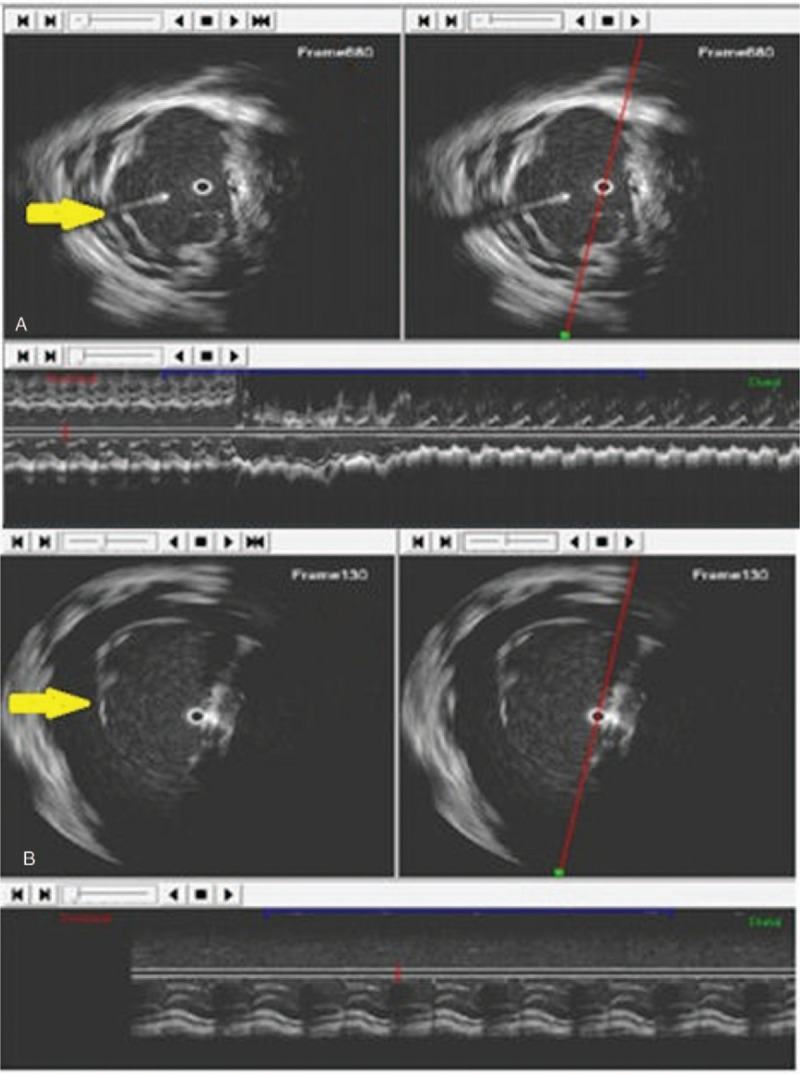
Intracardiac echography using a rotational probe. (A) The ViewFlex probe is inserted near the apex of the right ventricle. Please note the normal trabeculations which are present at the level of the lateral wall (yellow arrow). (B) The ViewFlex probe is inserted between the tricuspid valve and the apex of right ventricle. Please note the absence of normal trabeculations at the level of the lateral wall (yellow arrow). The regional absence of the trabeculations at the level of the “triangle of dysplasia” could not be seen with 2D echo and transesophageal echocardiography. 2D = 2-dimensional.
